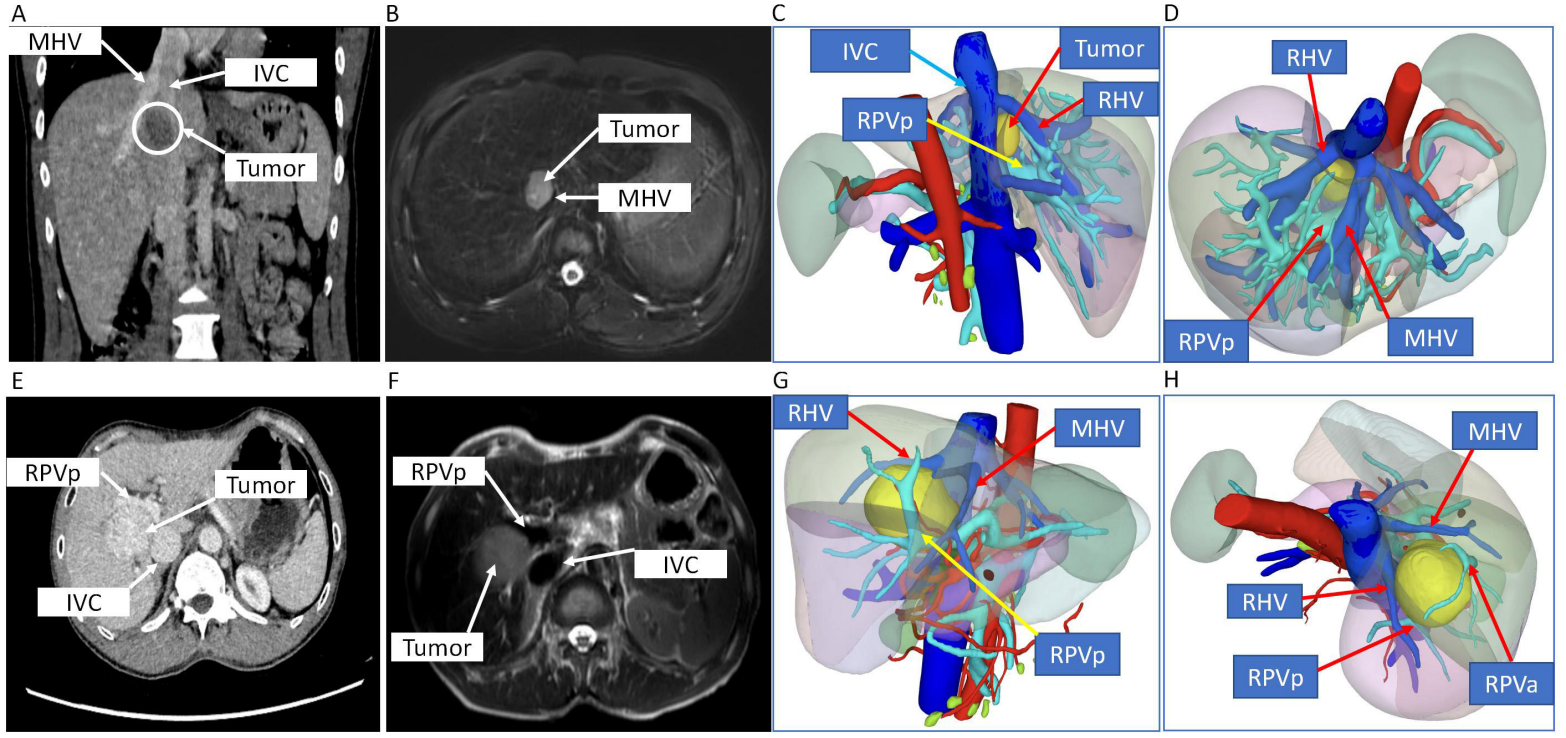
CT scan, MRI, and 3D digital modeling of liver imaging of the two patients. (A–D) Imaging examination of case 1. (A) Coronal position of CT scan of the tumor in the liver. (B) T2-weighted image of MRI of the tumor in the liver. (C, D) 3D digital modeling of liver and tumor. (E–H) Imaging examination of case 2. (E) Transverse position of CT scan of the tumor in the liver. (F) T2-weighted image of MRI of the tumor in the liver. (G, H) 3D digital modeling of liver and tumor. MHV, middle hepatic vein; IVC, inferior vena cava; RHV, right hepatic vein; RPVa, right anterior portal vein; RPVp, right posterior portal vein.
